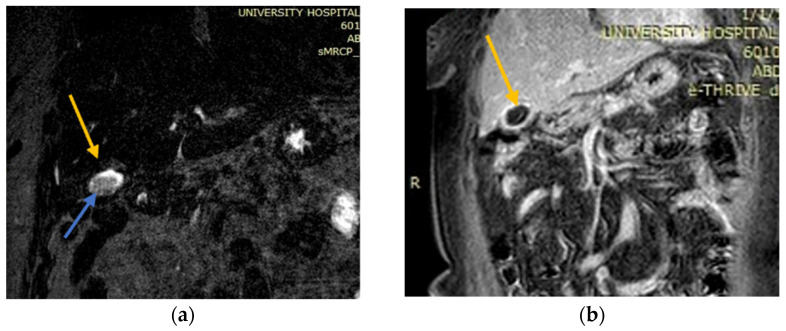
MRI images which showed findings consistent with acute cholecystitis. (a) Coronary MRI-image T1 post contrast of a gallbladder with diffuse mural thickening and contrast enhancement; (b) T2 MRI source image showing no perforation of the gallbladder. MRCP excluded choledocholithiasis and dilatation of the common bile duct. Yellow arrows: gallbladder; blue arrows: intraluminal stone and bile.
